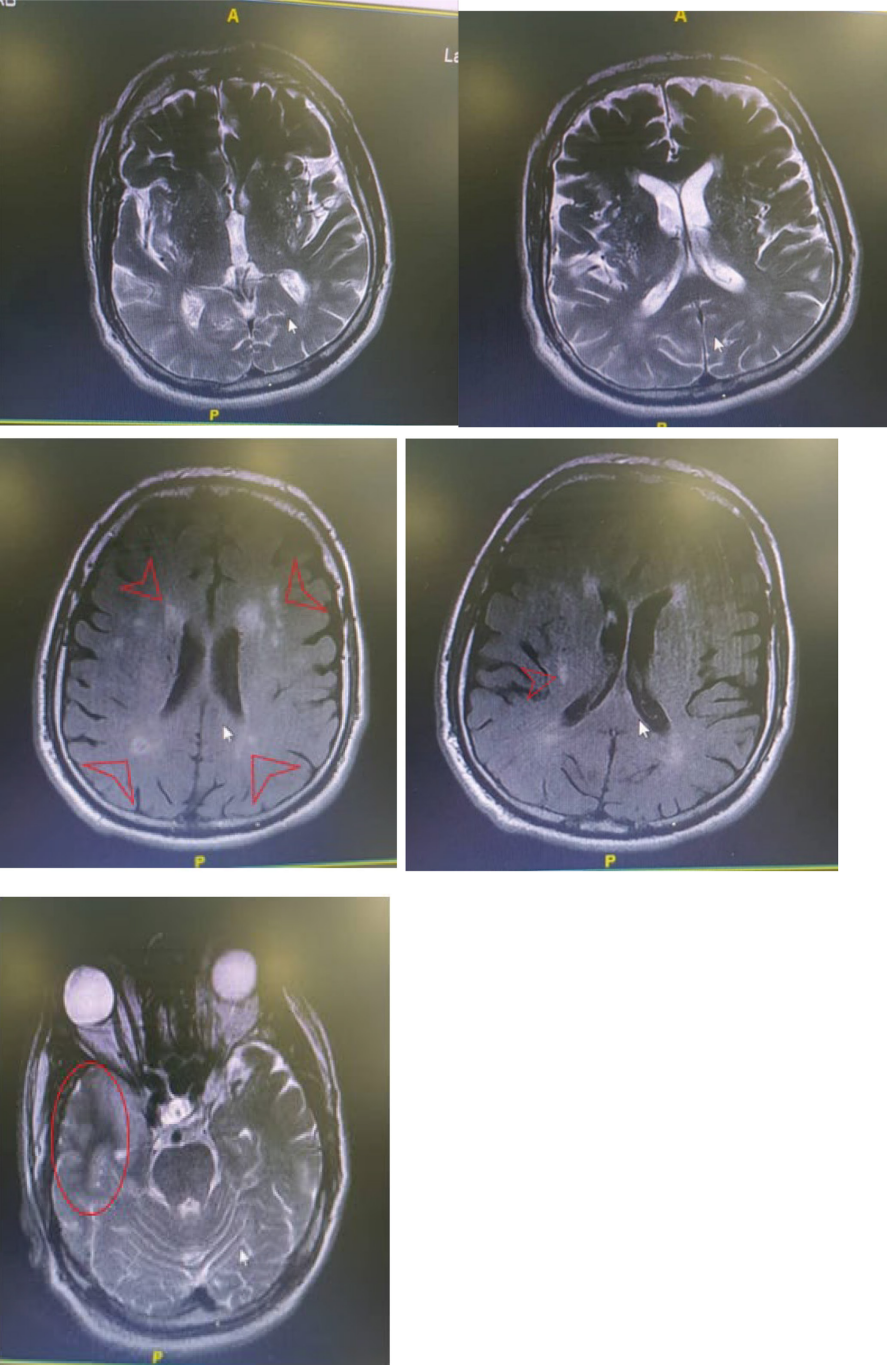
MRI of brain. Several T2, FLAIR hyperintense area is seen at deep white matter of both cerebral hemispheres in favor of deep white matter ischemic change (red arrows). Increased signal intensity with sulci effacement is seen at right temporal lobe involving medial and anterior temporal pole showing no post‐Gad enhancement in favor of encephalitis.
